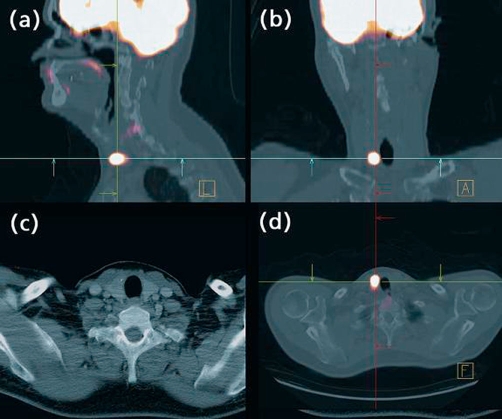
^18^FDG PET/CT showing the thyroid incidentaloma in sagital (a), coronal (b) and axial (d) view. Image (c) shows the right thyroid cyst on the CT scan.
